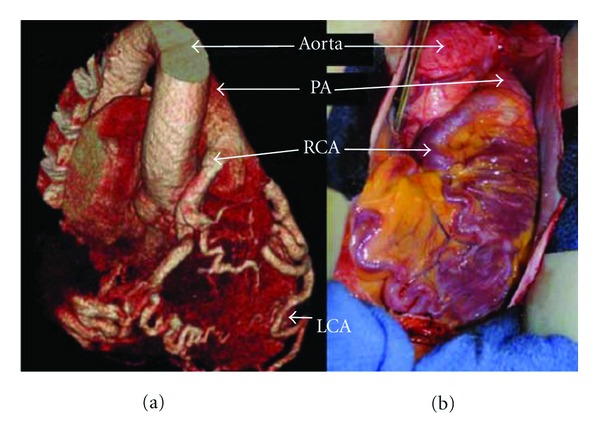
(a) Three-dimensional rendering of CT angiogram demonstrating ARCAPA. The right coronary artery originates from the anterior, lateral aspect of the pulmonary artery approximately 8 mm above the pulmonary valve. (b) intraoperative images of ARCAPA, prior to surgical correction. PA: pulmonary artery; RCA: right coronary artery; LCA: left coronary artery.
